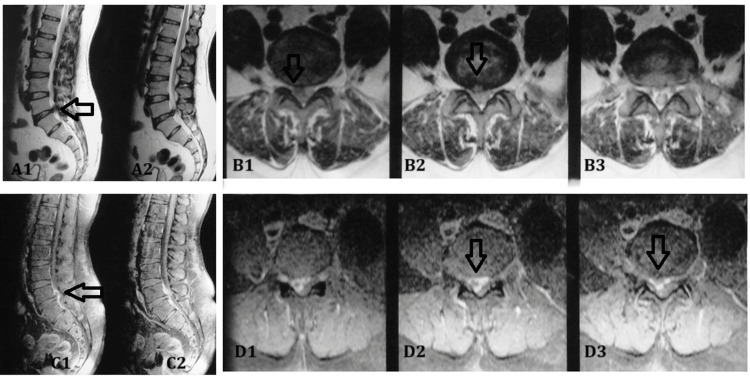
Sagittal (A1-A2) and axial (B1-B3) T2-weighted MRI at the L5-S1 level reveal a disc bulge resulting in the mild compression of the thecal sac; the areas of interest are marked by arrows in A1, B1, and B2. Corresponding contrast-enhanced sagittal (C1-C2) and axial (D1-D3) T1-weighted MRI do not demonstrate peripheral enhancement, suggesting an absence of active inflammatory or vascularized tissue response around the bulging disc; arrows highlight the areas of interest in C1, D2, and D3. A1, B1, B2 (T2-weighted images): arrows indicate the location of the disc bulge at L5-S1, associated with mild compression of the thecal sac. C1, D2, D3 (post-contrast T1-weighted images): arrows highlight the same disc region, where no peripheral enhancement is observed, supporting the absence of active inflammation or granulation tissue. MRI: magnetic resonance imaging
